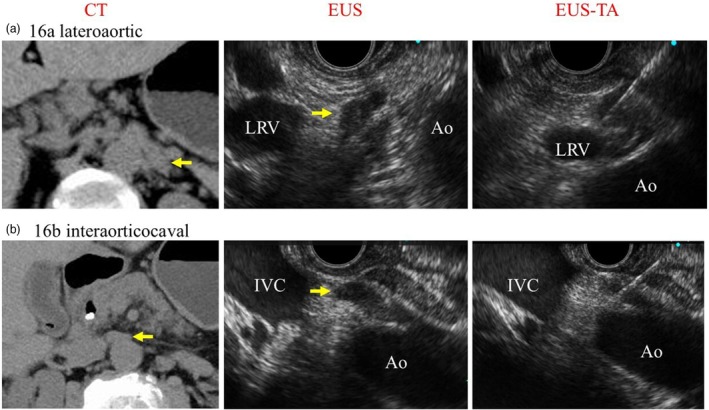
Computed tomograph (CT) and endoscopic ultrasound‐guided tissue acquisition (EUS‐TA) images of para‐aortic lymph nodes. Ao, aorta; EUS, endoscopic ultrasound; IVC, inferior vena cava; LRV, left renal vein. (a) 16a lateroaortic observation from the stomach. (b) 16b interaorticocaval observation from the descending segment.
